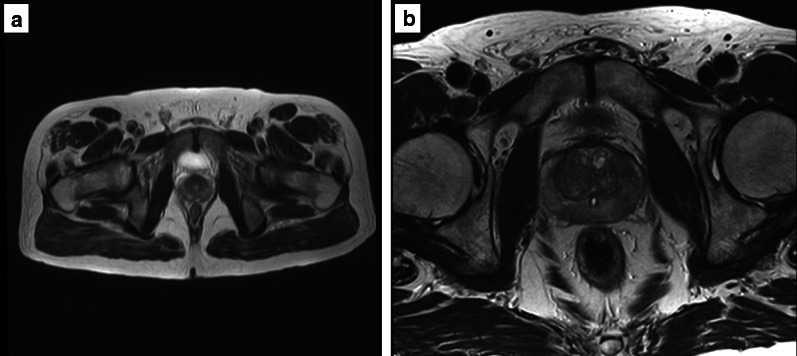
T2-weighted axial images of a suboptimal (41 × 42 cm) (a) and optimal (17 × 18 cm) (b) field of view according to the PI-RADS v.2.1 guidelines
